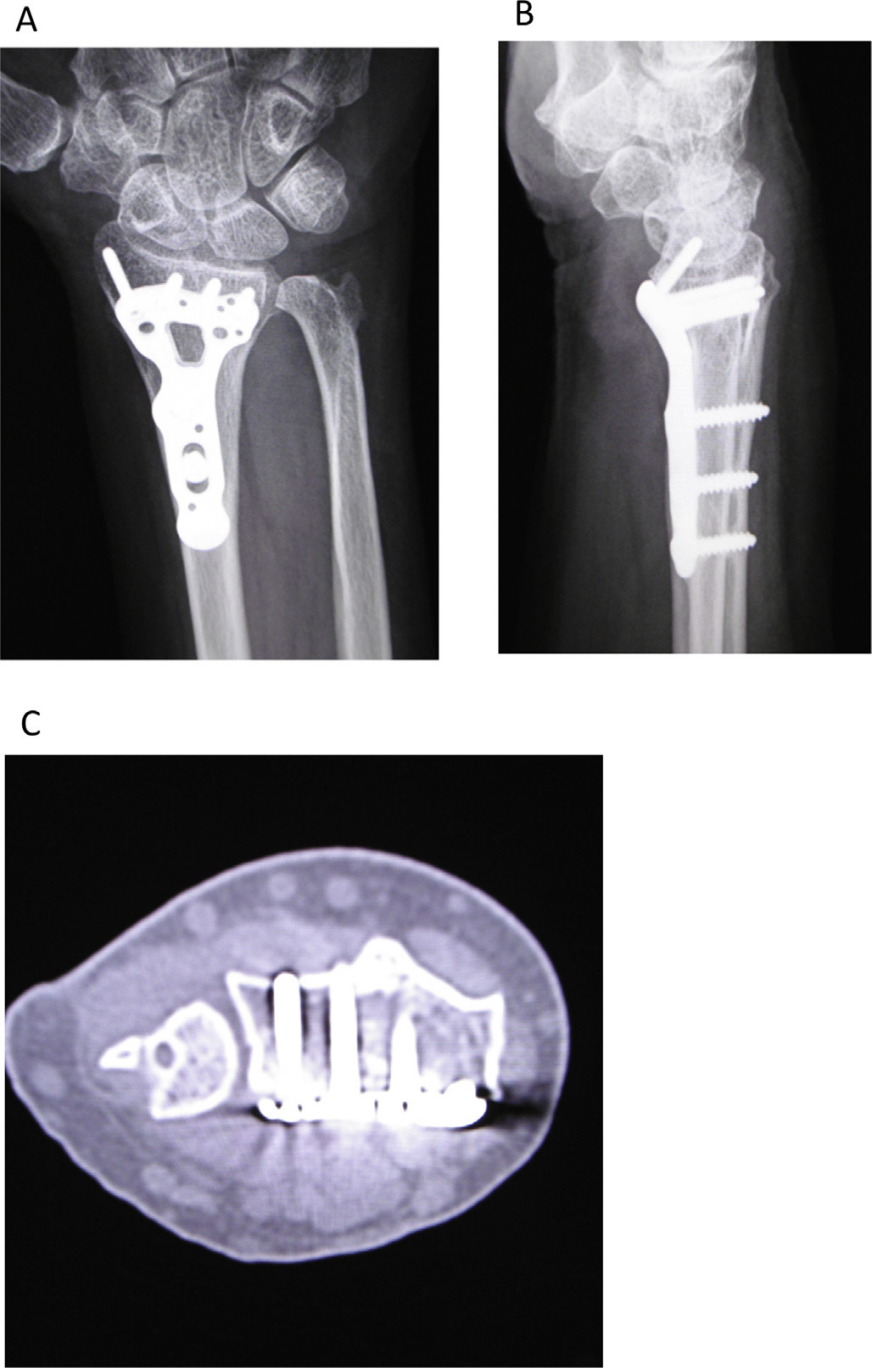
Plain radiography and CT 2 years after surgery. On plain radiography, congruency of DRUJ was favorable (A: frontal view, B: lateral view). With an axial view on CT, the burr hole in the suture anchor insertion site in the ulnar fovea was dilated (C).
